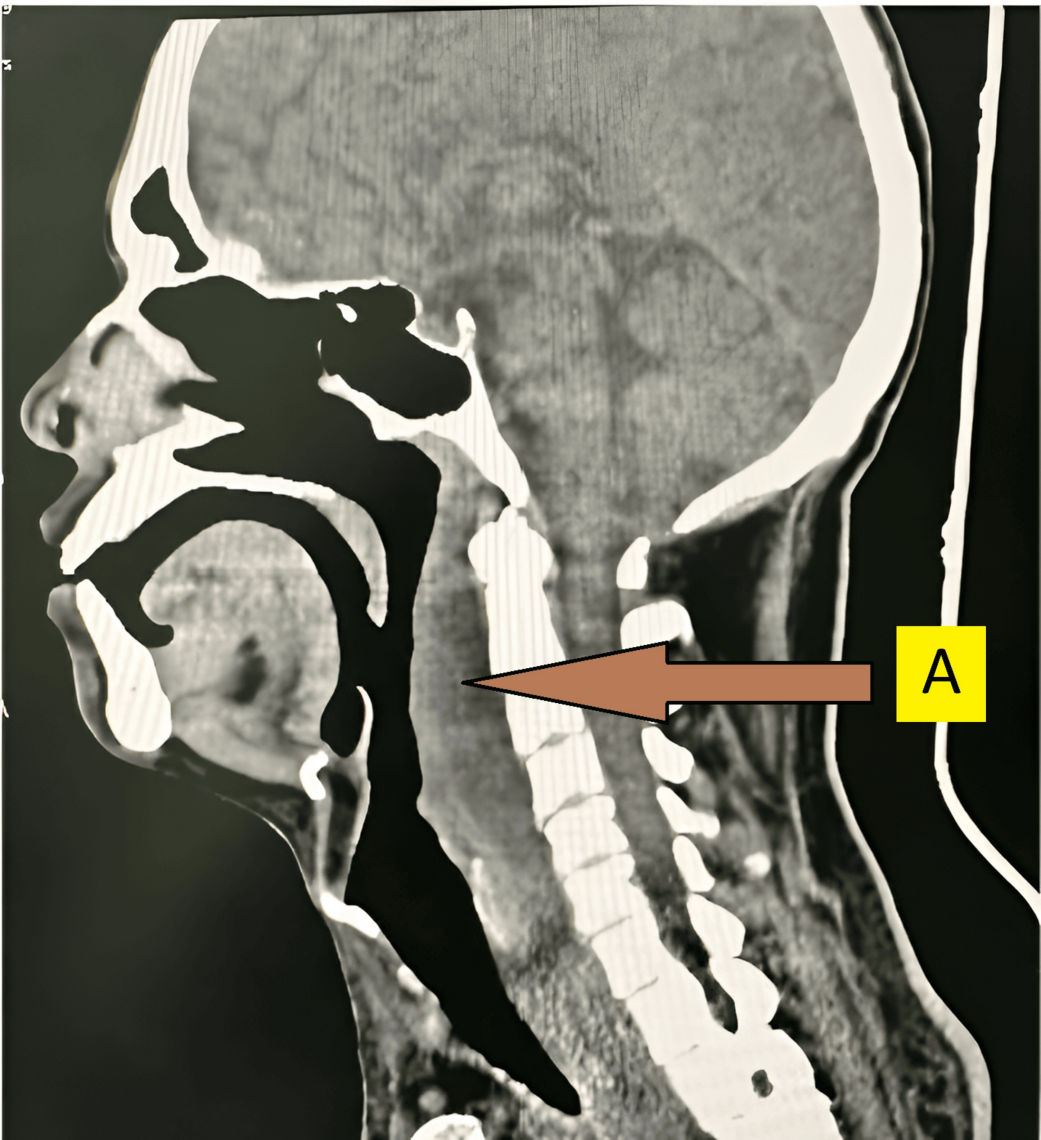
CT of the neck revealed a retropharyngeal abscess. A: Retropharyngeal abscess.
